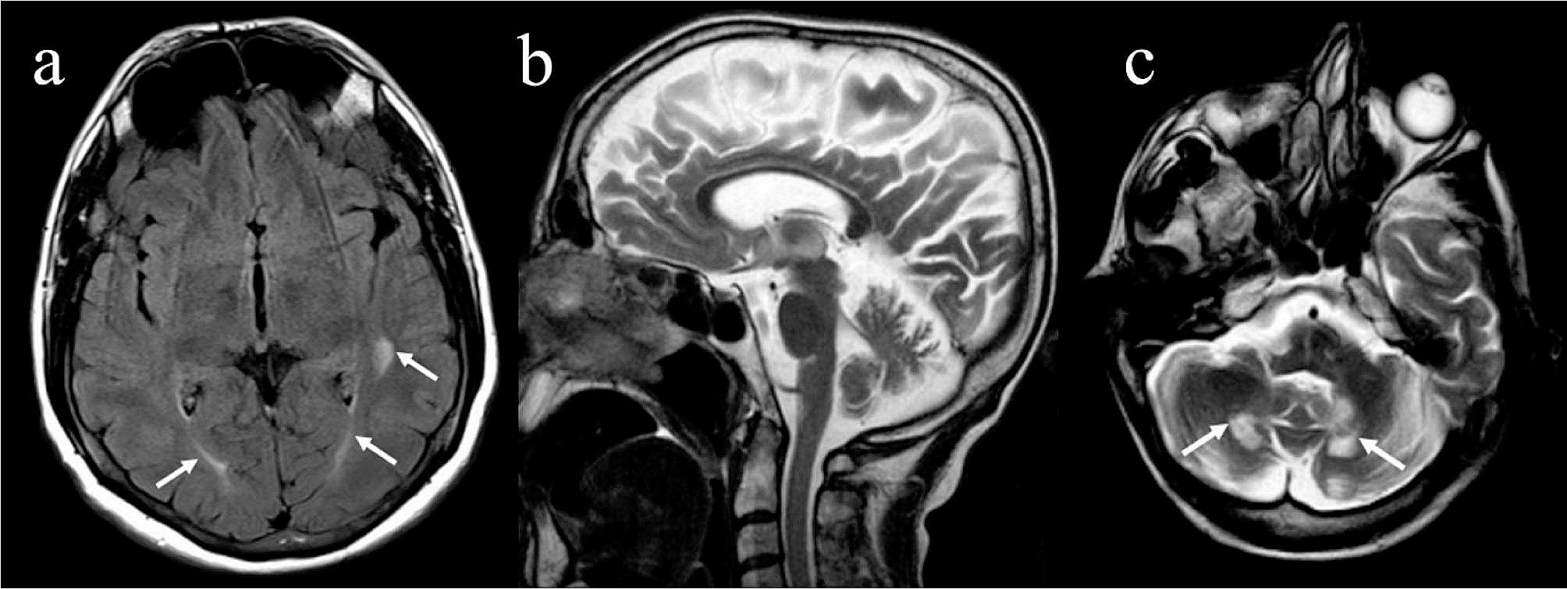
Axial FLAIR MR image (patient 7), at the level of thirth ventricule of the same patient revealed bilateral periventricular hyperintensity (a) (white arrows), sagittal (b) and axial (c) T2-weighted MR images show cerebral and cerebellar atrophy with prominence of the sulci and hyperintensity of the bilateral dentate nuclei (arrows)
